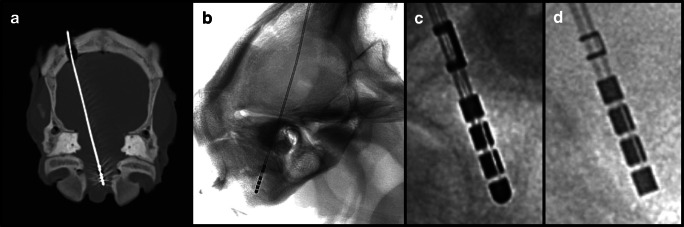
Imaging modalities in this modal trial. a CT scan after electrode implantation with maximum intensity projection showing a transparenchymal course of the electrode (Cartesia™). b Lateral projection (note the overlap of the meatus acusticus externus) of the 3D-RF with Cartesia™ implanted. c Excerpt from the 3D-RF, which depicts the so-called iron-sight sign of a Cartesia™ electrode. This occurs exactly orthogonal to the electrodes orientation—note the c-shaped lead marker. d “Iron-sight” sign of an Infinity™ electrode
